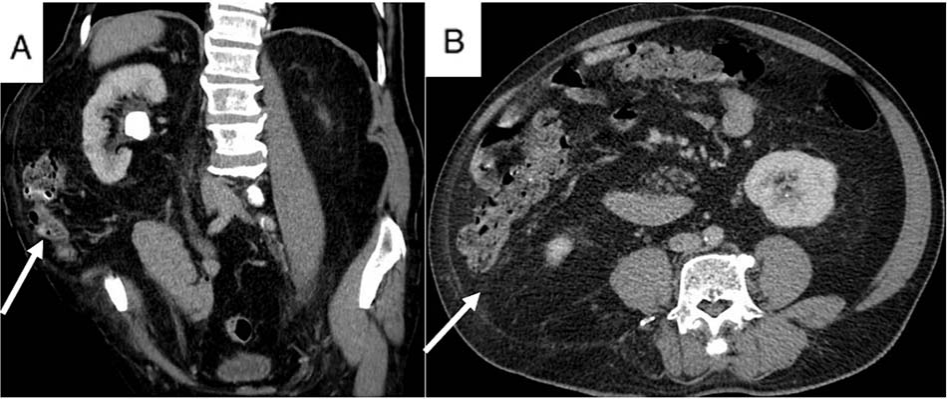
Patient’s CT scan shows right UPJ stone (A) and lumbar hernia with mesentery inside (A and B, arrow).
